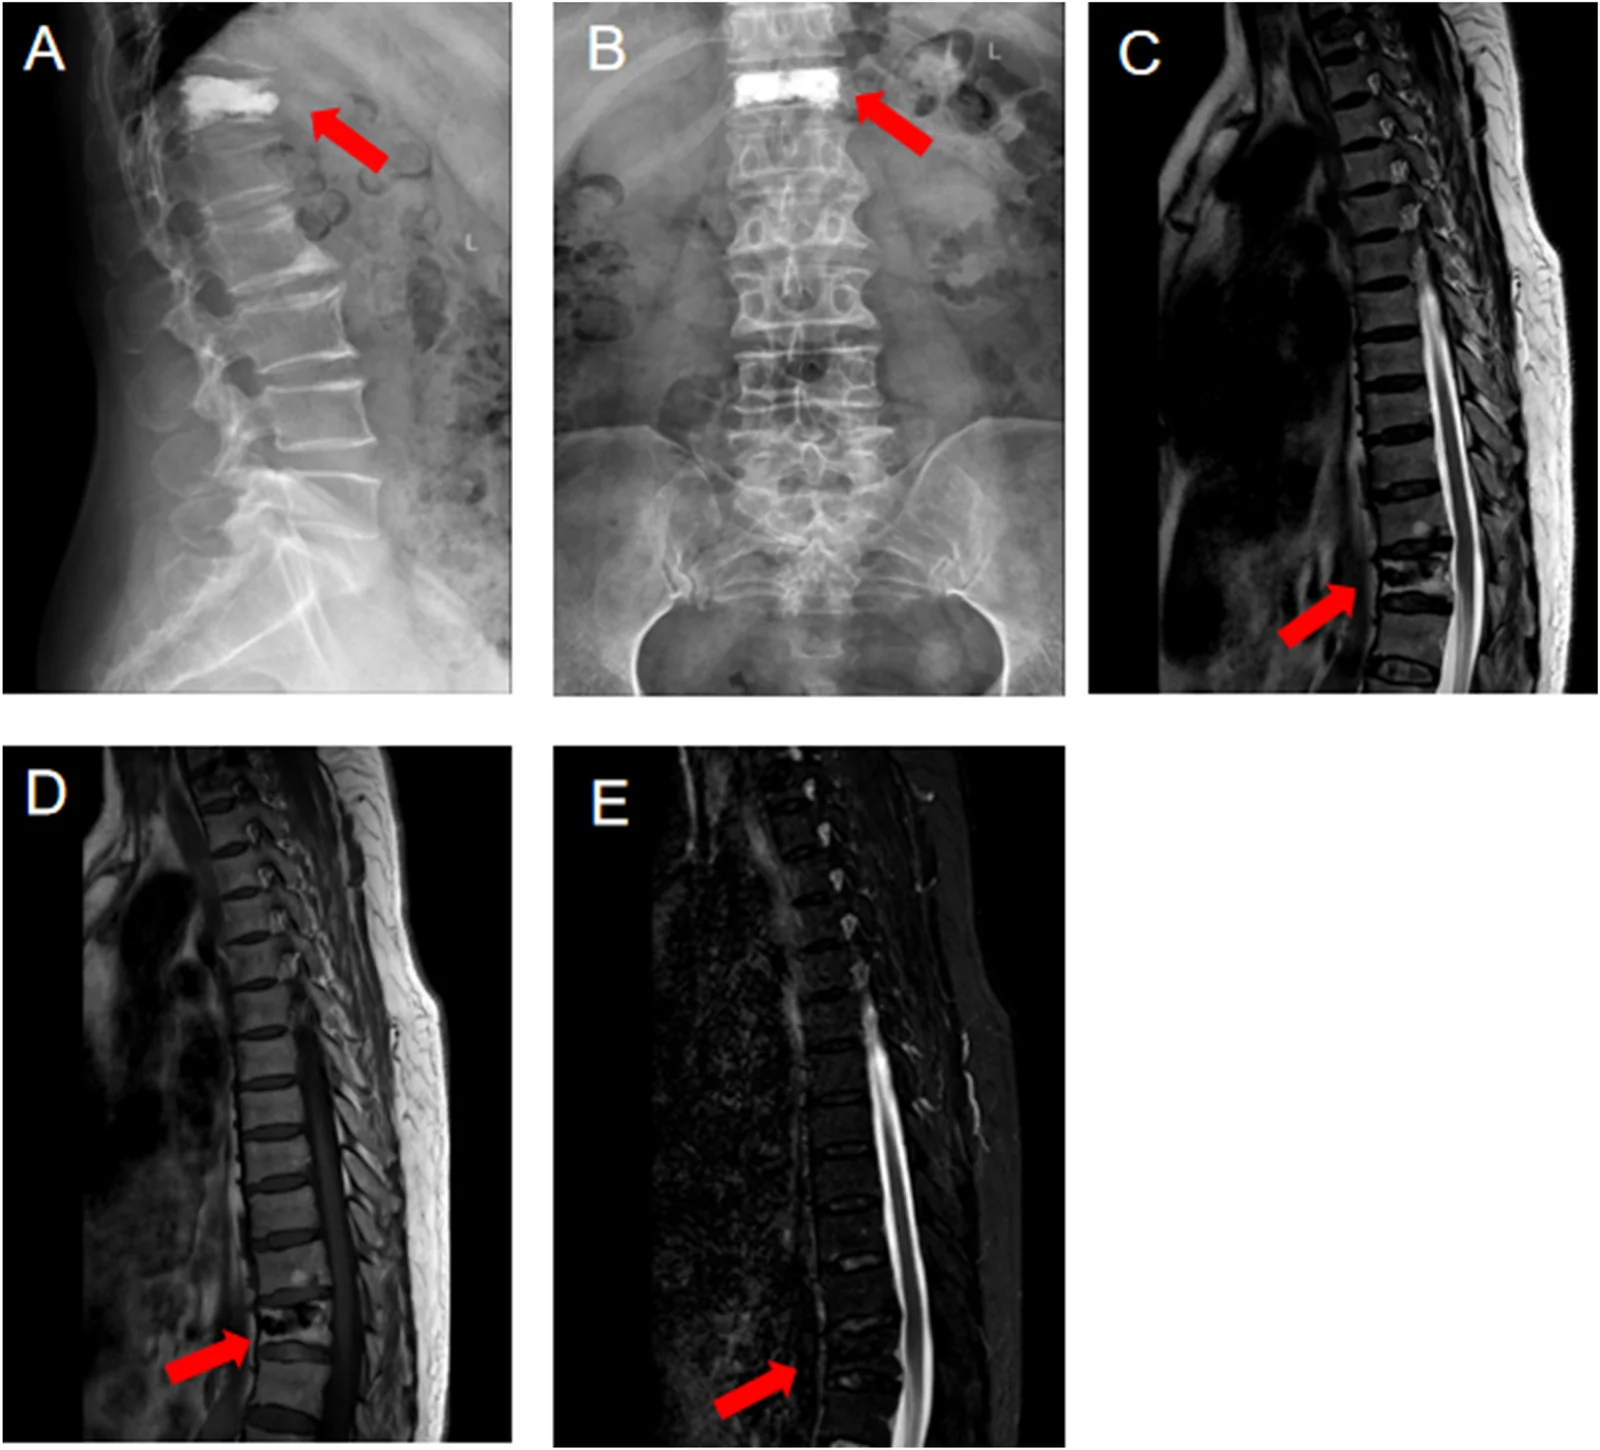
Three-month follow-up plain radiography and MRI images (A–E) demonstrating significant restoration of vertebral height and progressive bony healing of the T12 compression fracture.
